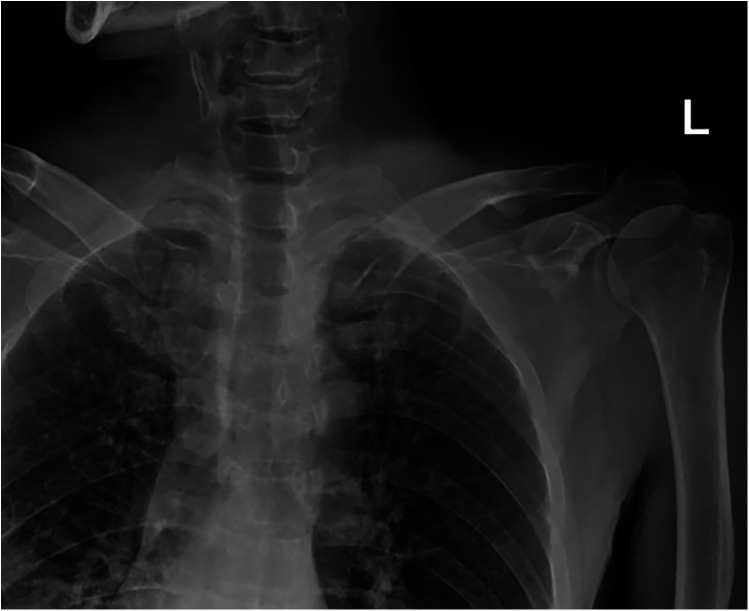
Anterior-posterior clavicle radiograph showing fresh bilateral fractures of medial clavicle.
